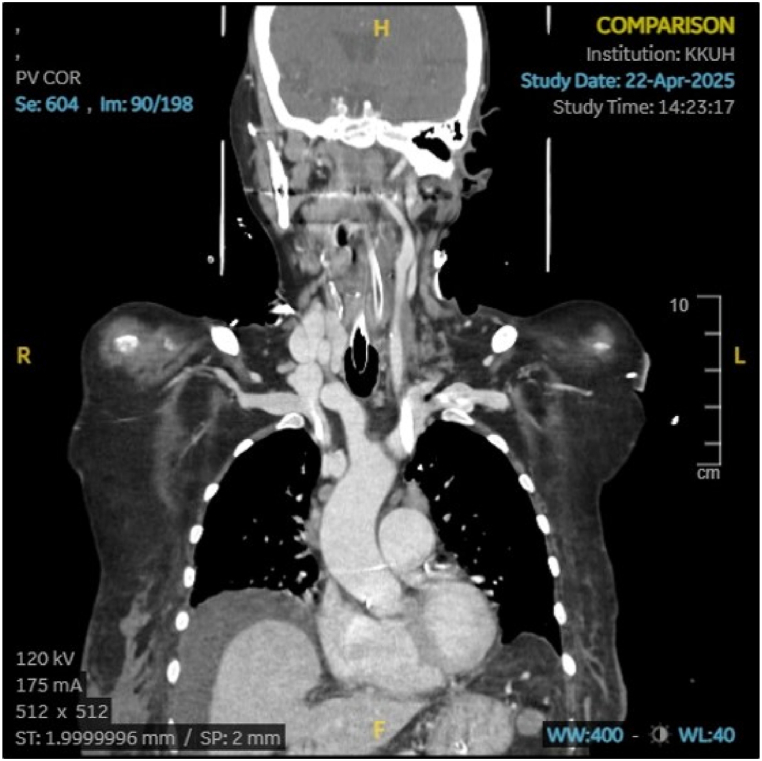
CT angiography coronal image of the chest and neck depict that the dialysis catheter traversed the left internal jugular vein into the left innominate vein, then deviated extraluminally through the left mediastinum before terminating within the left lower pulmonary vein.
